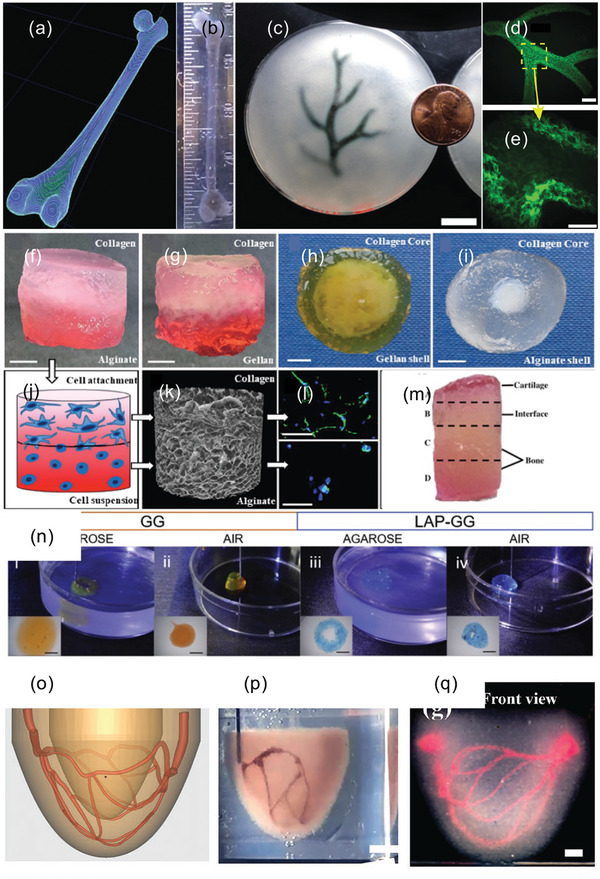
a) A model of a human femur from 3D CT imaging data processed for FRESH printing and b) printed in alginate. c) Human right coronary arterial tree from 3D MRI is processed and FRESH‐printed tree in alginate (black) and embedded in the gelatin slurry support bath. d) Fluorescent alginate (green) of the arterial trees printed in (e) imaged in 3D to show the hollow lumen and multiple branching. Scale bars, (c) 10 mm, (d) 2.5 mm, (e) 1 mm. Reproduced (Adapted) under the terms of the CC0 license.^[^
